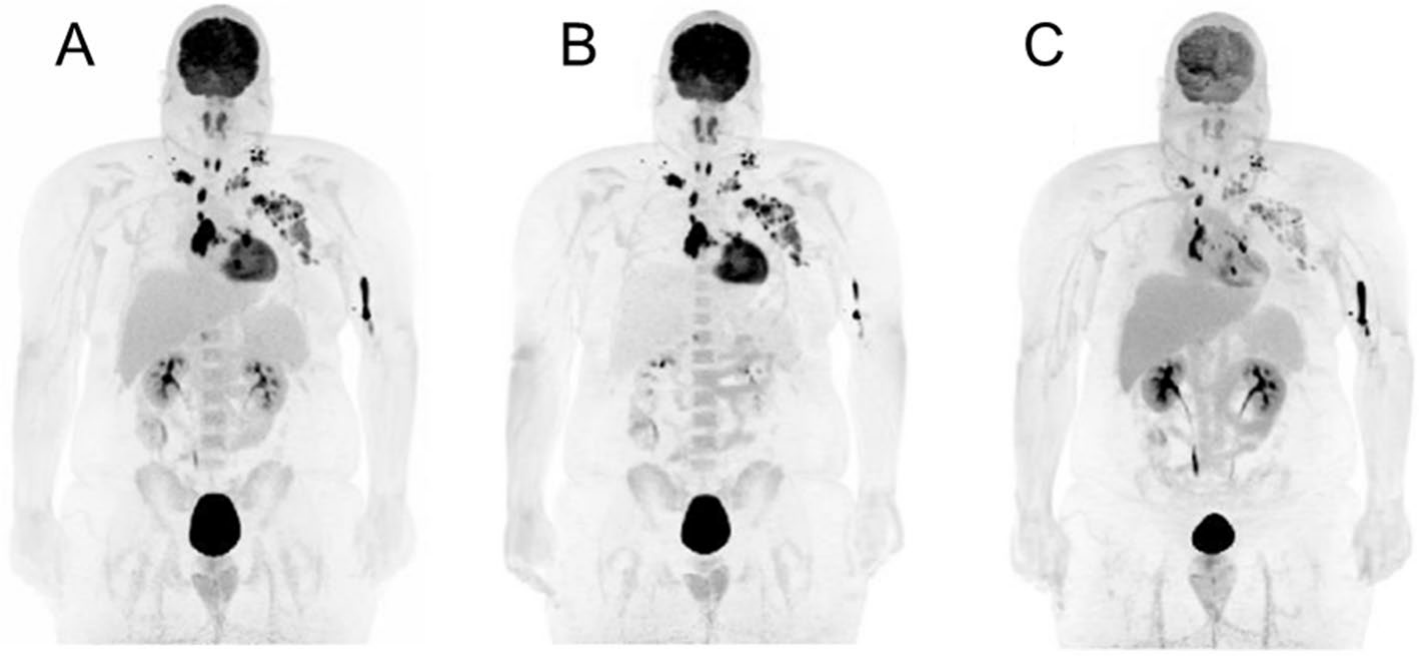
Example static SUV map for a patient with Lymphoma at 2-[^18^F]FDG PET/CT (A), Patlak K_i_ map demonstrating metabolically trapped FDG (B) and Patlak distribution volume (DV) showing non-trapped FDG (C)
